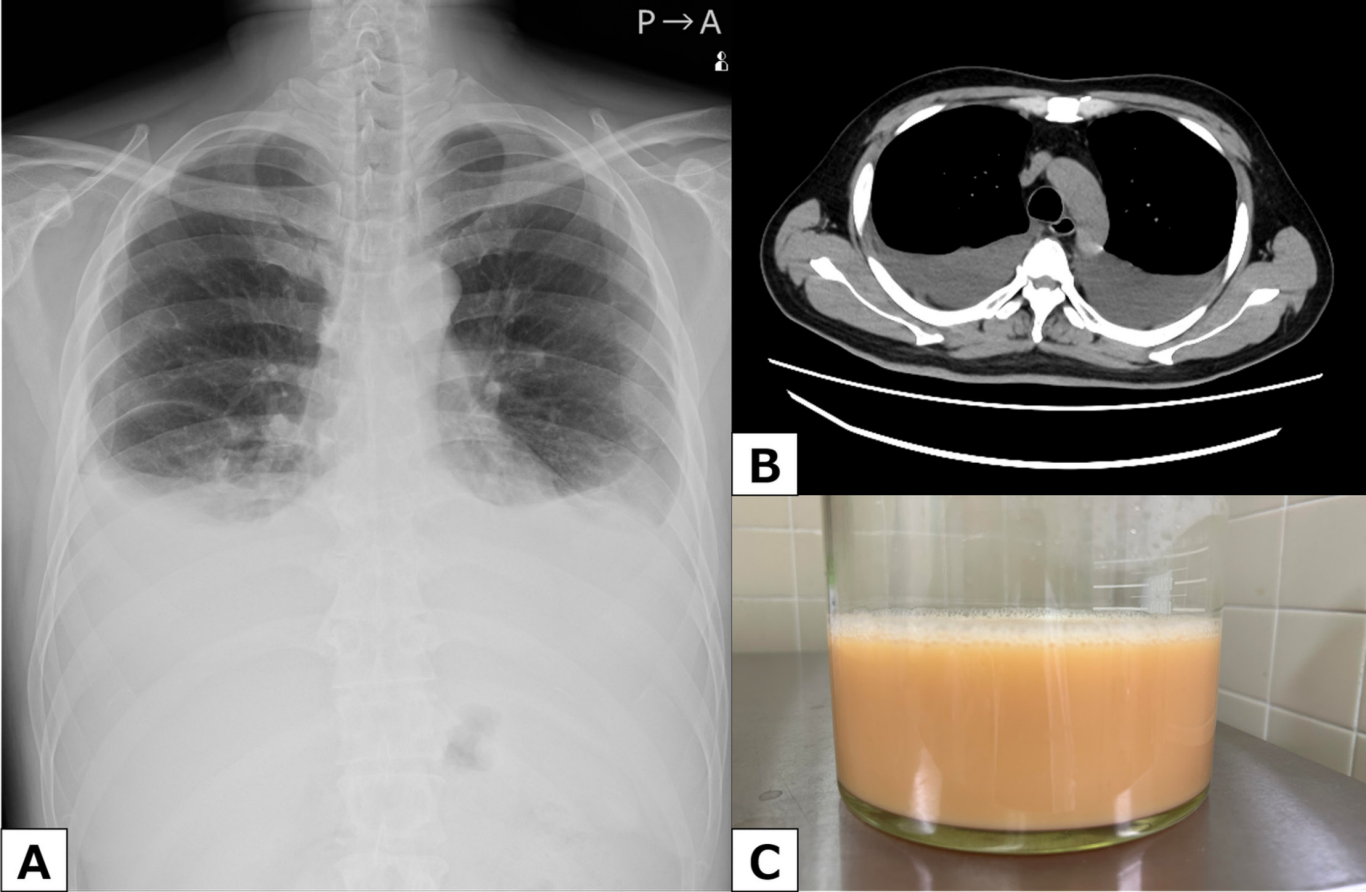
Radiological and thoracentesis findings at initial presentation. (A) Chest radiograph obtained at a routine outpatient visit showing marked bilateral pleural effusions. (B) Computed tomography (CT) also demonstrated marked bilateral pleural effusions, with no evidence of tumours or other structural lesions affecting the thoracic duct. (C) Thoracentesis of the right pleural cavity yielded milky pleural fluid.
